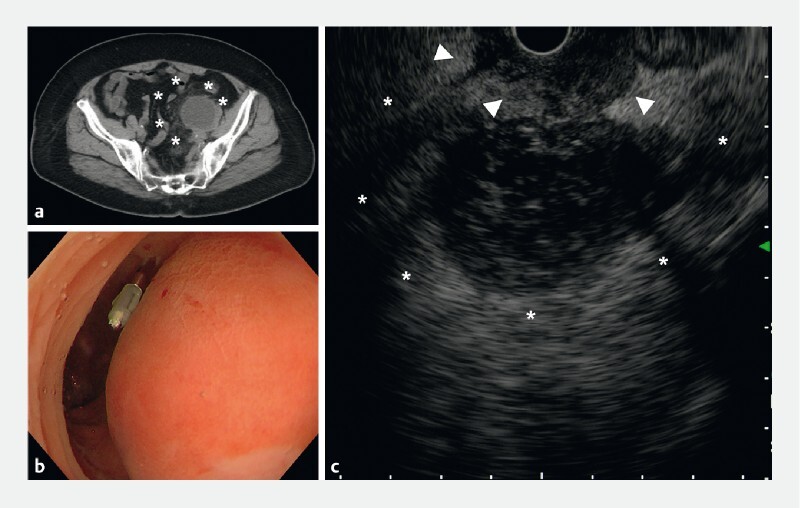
Computed tomography revealed an infected lymphocele after an operation for ovarian cancer.
